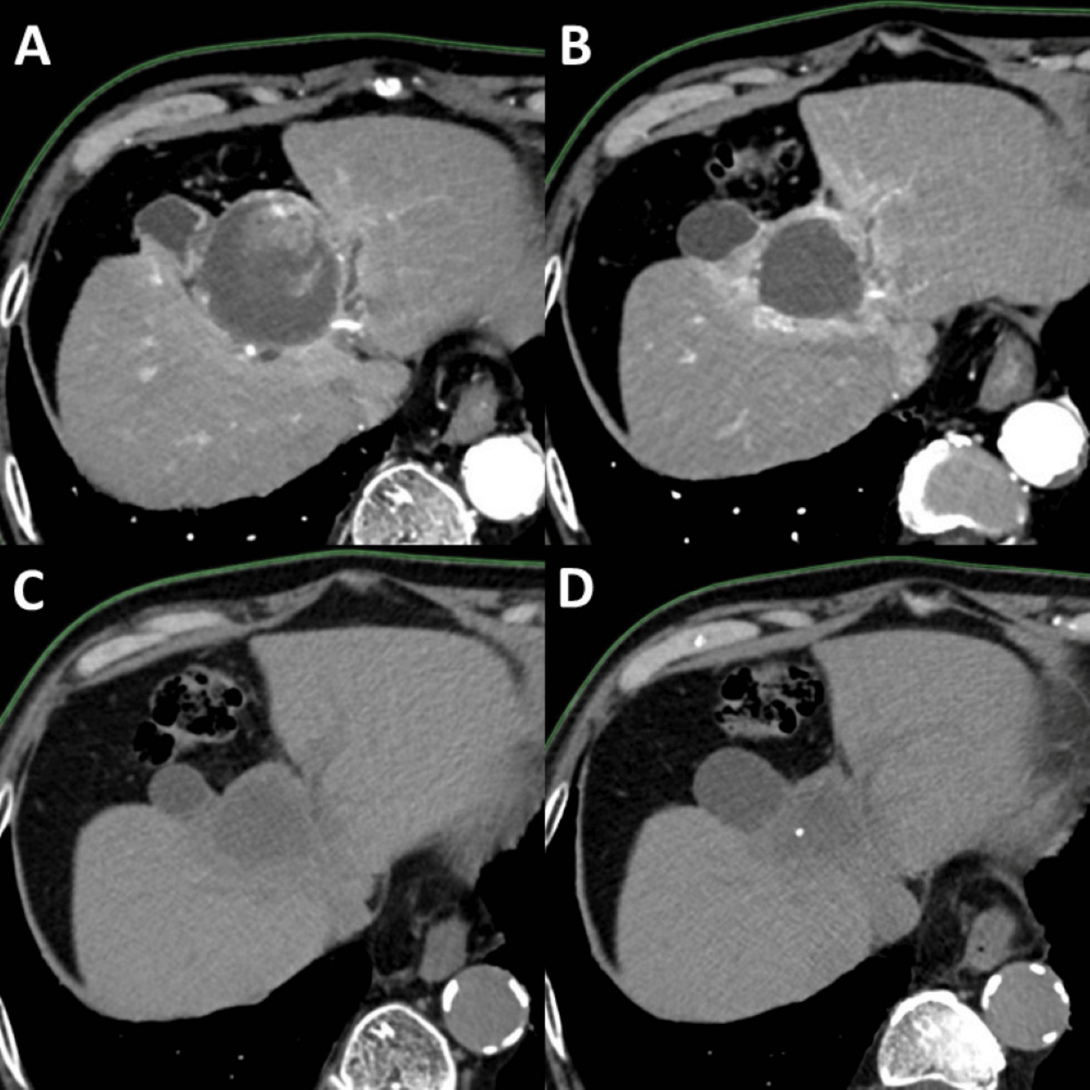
Follow-up CT scans after SBRT The solid tumor component decreased in size at 11 months after the first-stage SBRT (A) and had disappeared by 21 months (B). A complete response was maintained at 32 months (C) and 44 months (D) following the first-stage SBRT. SBRT, stereotactic body radiotherapy
